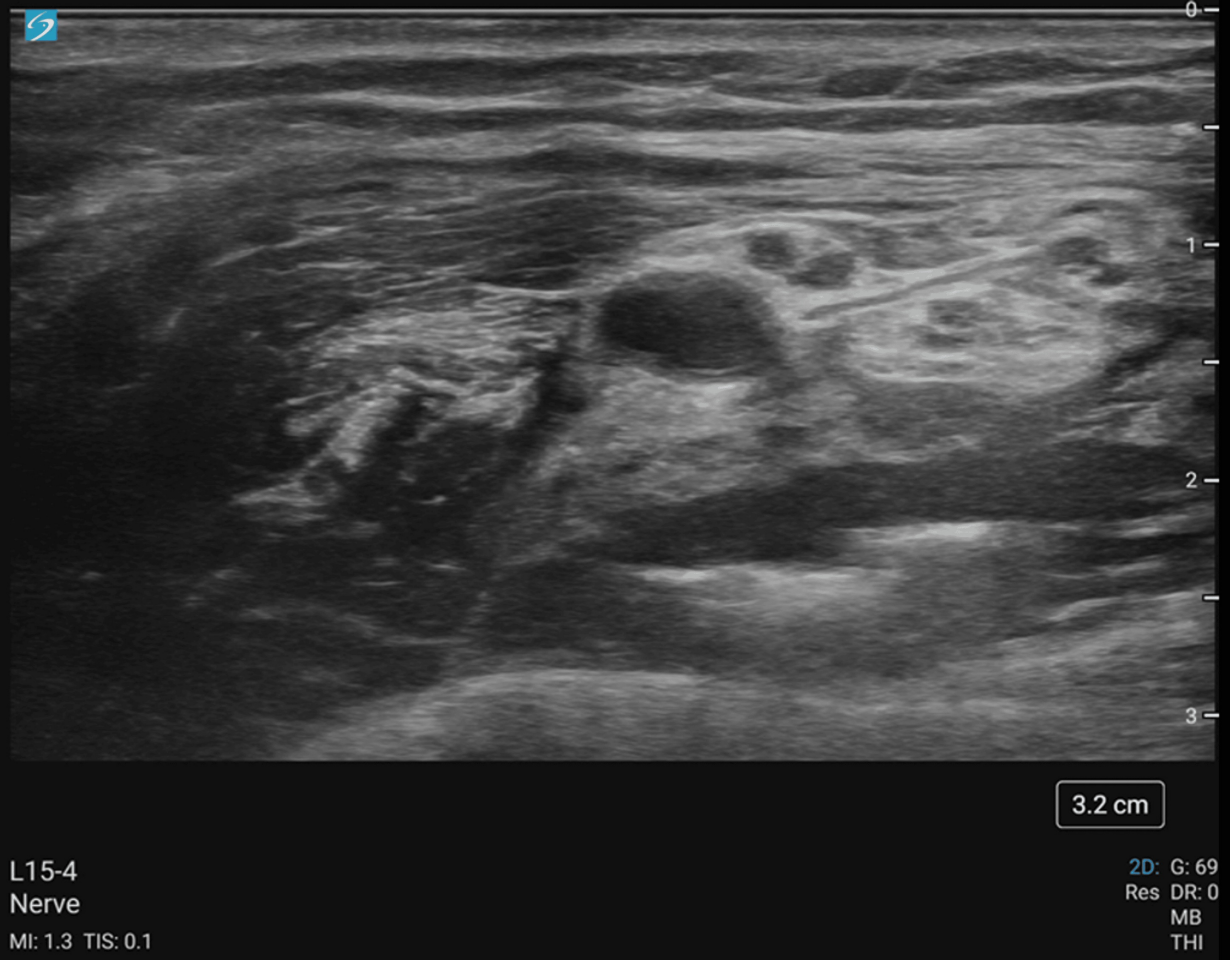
Ultrasound-guided axillary nerve block on the patient with a 15-4 MHz linear transducer and a 20-gauge, 4-inch echogenic B. Braun Ultraplex 360® needle
